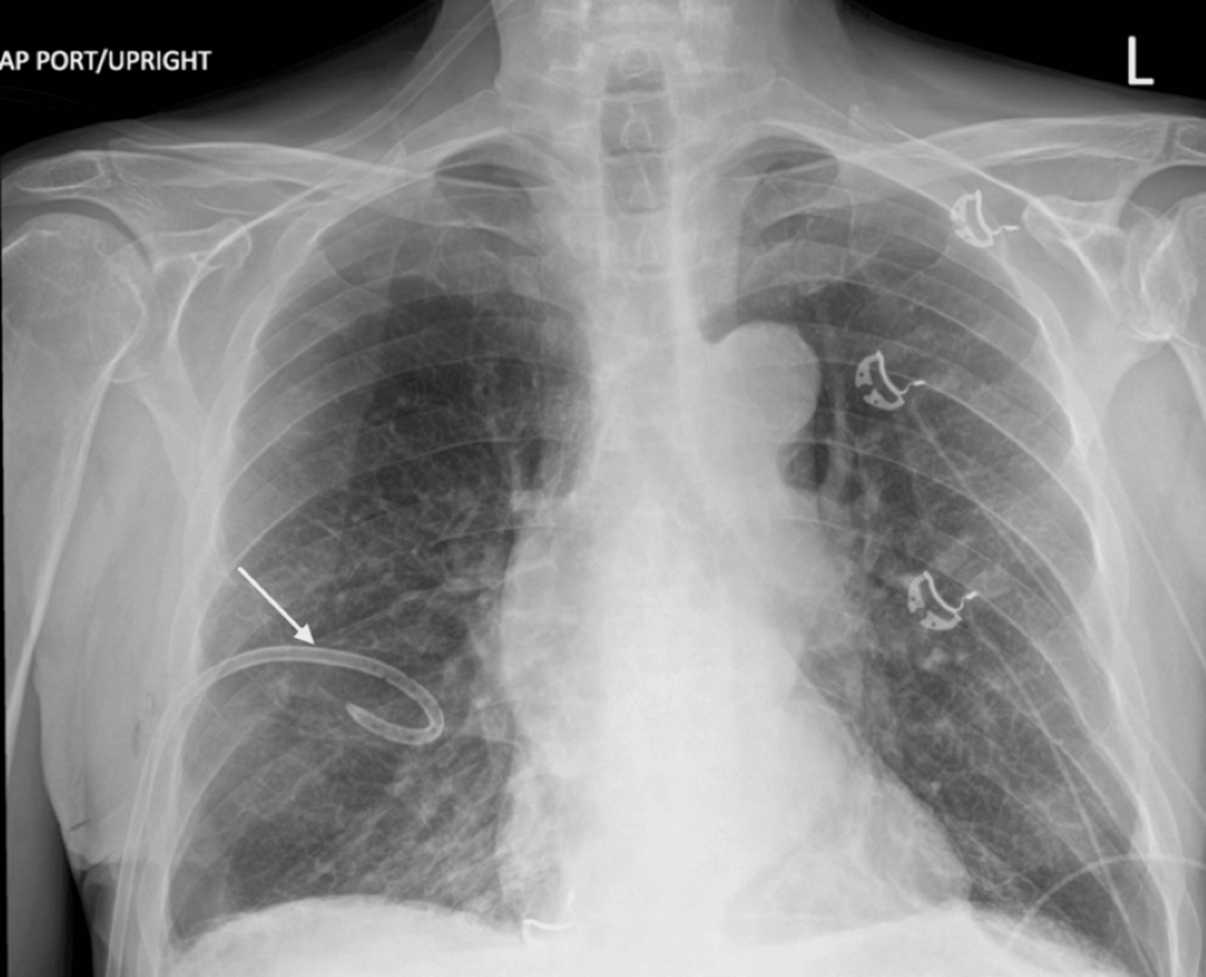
Chest X-ray showing near-complete resolution of pneumothorax after chest tube placement, with underlying emphysematous changes
